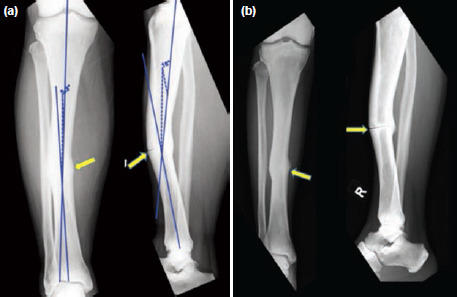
Pre-operative radiographs. (a) AP and lateral radiographs showing a fracture of the midshaft of the right tibia. The yellow arrow indicates the fracture site. The blue lines depict the angular deformities. On the AP radiograph, a varus angulation of 5.3° is seen. On the lateral radiograph, a procurvatum deformity of 16° is seen. (b) AP and lateral radiographs after nine months of follow-up showing non-union of the midshaft of tibia.
